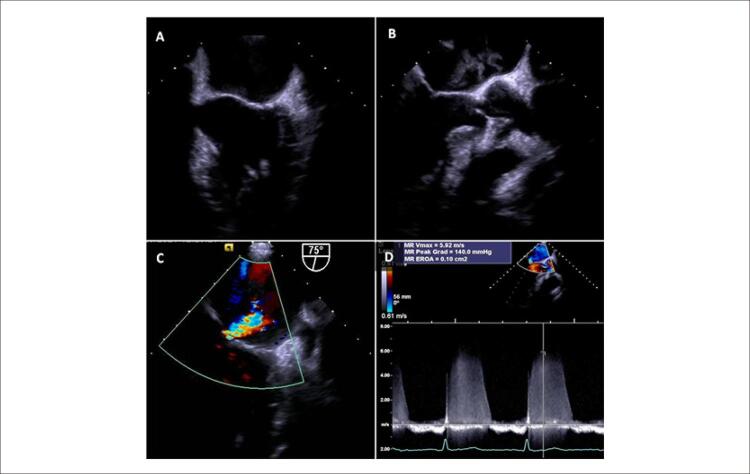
Ecocardiografia transesofágica realizada no 51º dia de internação após antibioticoterapia. Revela eliminação completa da vegetação valvar mitral (visão zoom da valva mitral em 60º (A) e 120º (B)). Doppler colorido (C) e de onda contínua (D) revelando regurgitação valvar mitral leve (área efetiva do orifício regurgitante de 0,10 cm 2 pelo método PISA).
